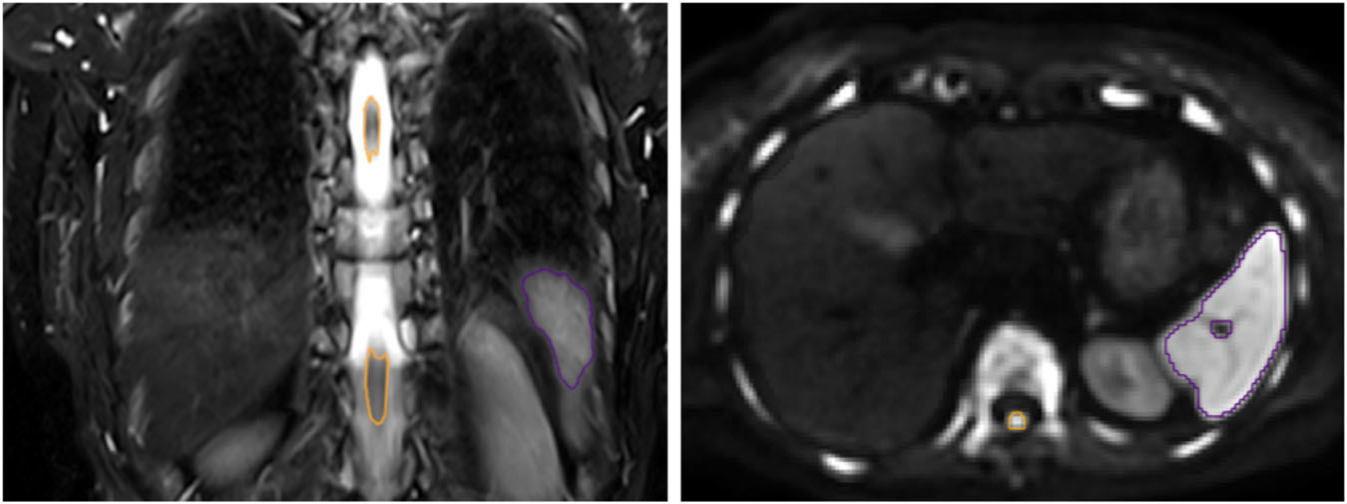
Examples of segmentations of the spleen (violet) and the spinal cord (orange) on coronal T2-TSE (right) and axial DWI (b800, left)
